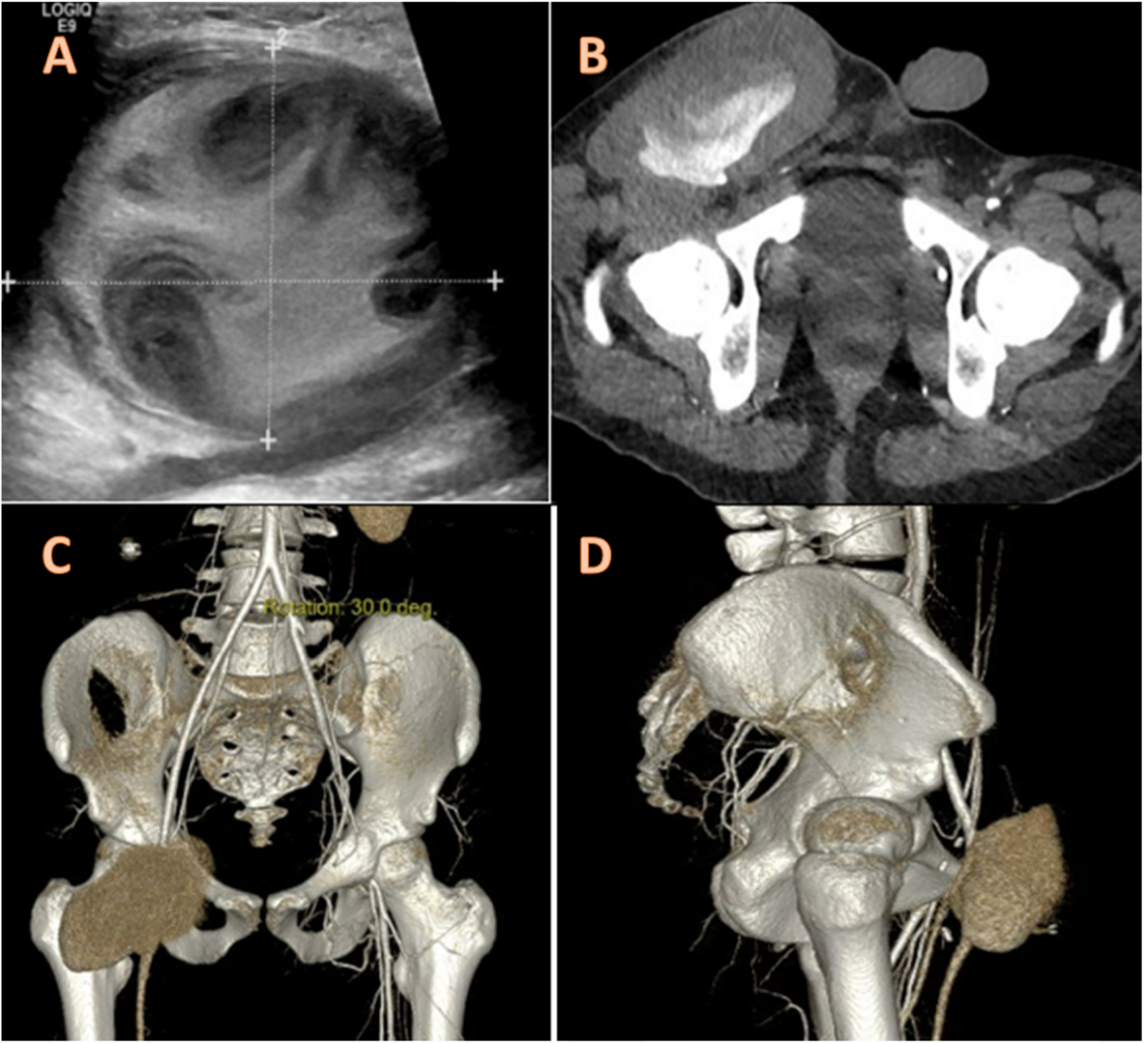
A 21-year-old male patient developed acute right groin swelling after right femoral ECMO decannulation. Groin US revealed a large swelling (7 × 5 cm) with heterogenous echogenicity in the right groin area arising from right femoral artery with the neck measuring 0.6 cm (a). CT angiography revealed the large pseudo-aneurysm (12 × 6 cm) from the right common femoral artery with active contrast extravasation (b). Multiplanar reconstruction and advanced 3-D postprocessing were performed and revealed the large pseudo-aneurysm with good distal arterial flow (c, d). Vascular surgery was done with evacuation of the pseudo-aneurysm and repair of the femoral artery
